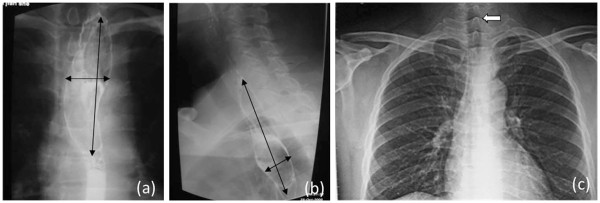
Barium meal (baM) examination of esophagus showing significant broadening in the cervical segment. (a) Front view (b) Side view. (c, arrow) X-ray of the chest showed that the trachea leaned to the right.
